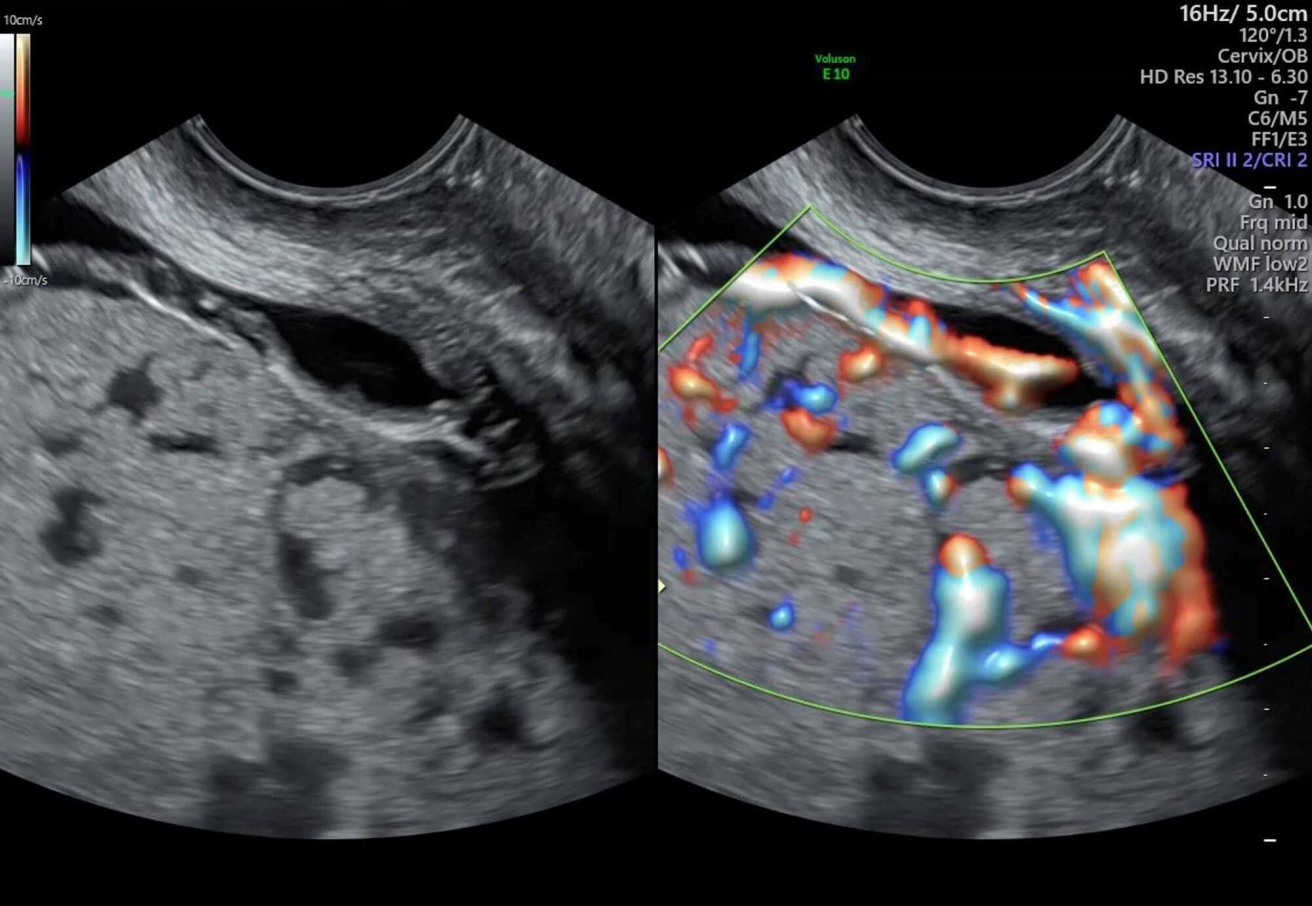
Transabdominal ultrasound image displaying engorged vessels extending along the junction of the bladder with the placenta and uterus. The vessels appear to extend into the bladder, raising suspicion for placenta percreta
